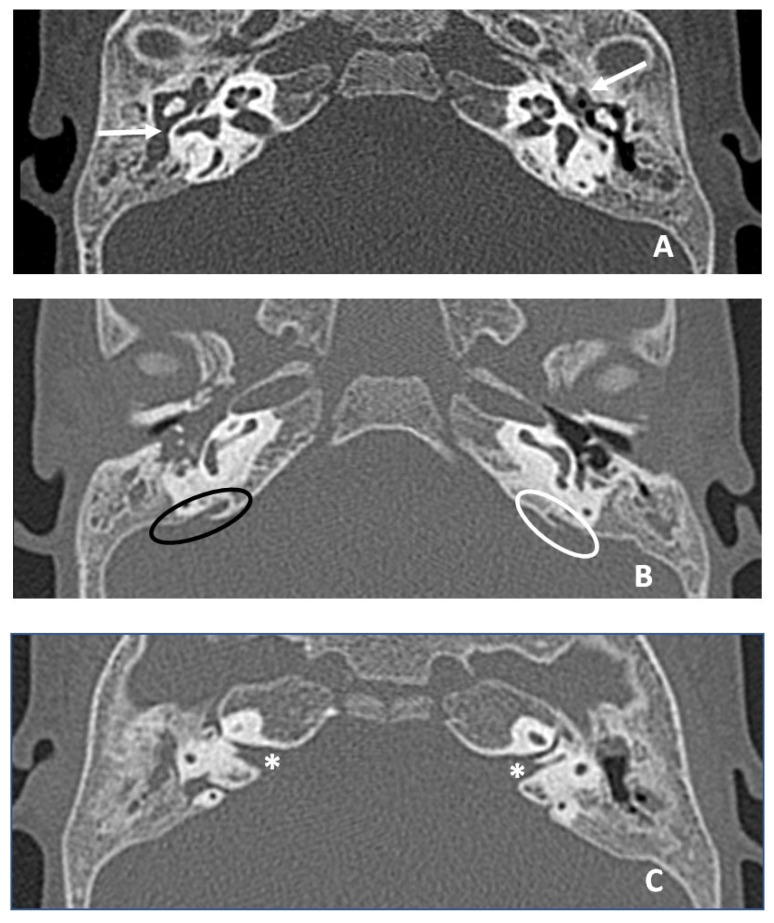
CT images in axial plane. (A) White arrows indicate hypodense material in middle ear and mastoid compatible with otitis media with effusion, greater on the right. (B) Ovals indicate the vestibular aqueduct bilaterally, black oval on the right (slightly enlarged aqueduct, larger than the diameter of posterior semicircular canal) and white oval on the left. (C) White asterisks indicate Bill bar bilaterally, thickened and more developed in length than normal.
